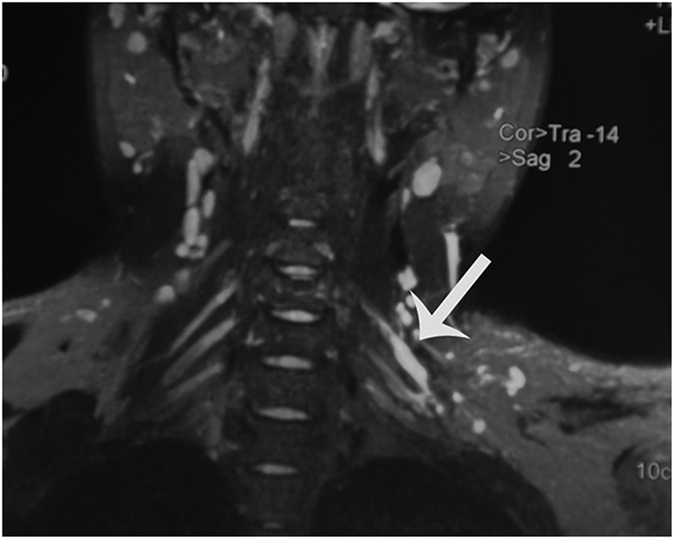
A 25-year-old man with a history of motor cycle accident nine months back. Coronal image shows C5 root is continuous but thickening in T2-weighted images.
